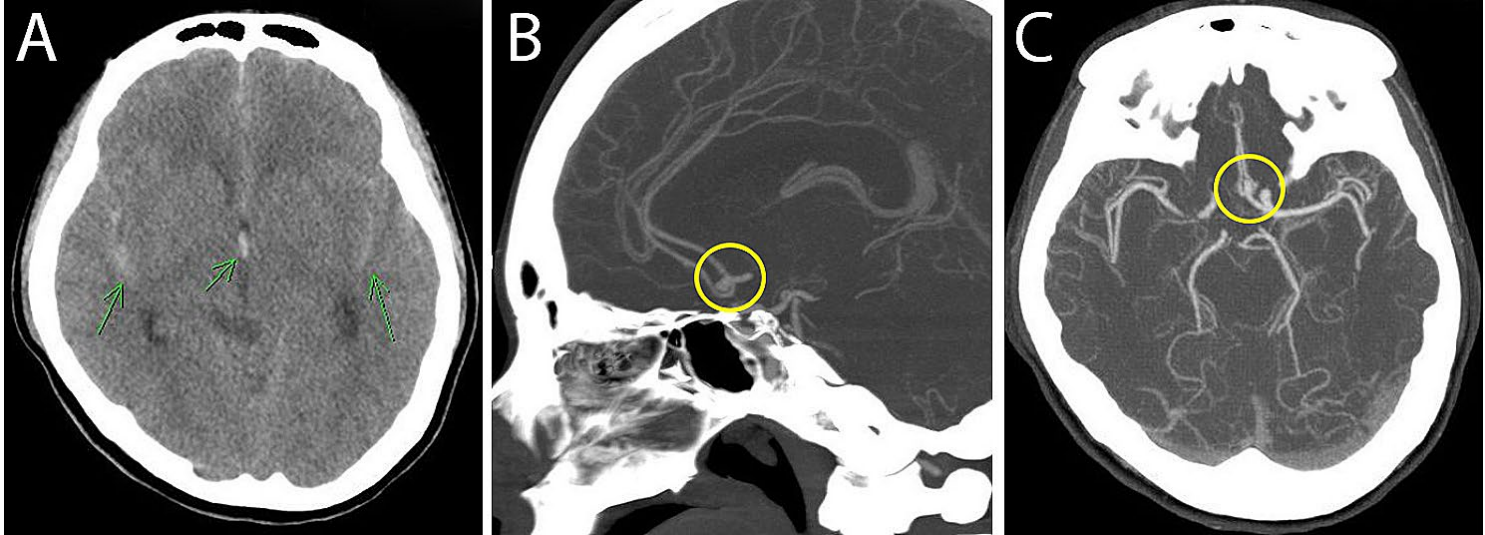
Brain computed tomography (CT). (A) Brain CT revealed a diffuse subarachnoid hemorrhage (green arrows). (B&C) The CT angiography also showed a small aneurysm with lobulated contour, 4.6 mm in dimension noted in anterior communicating artery (yellow circles)
